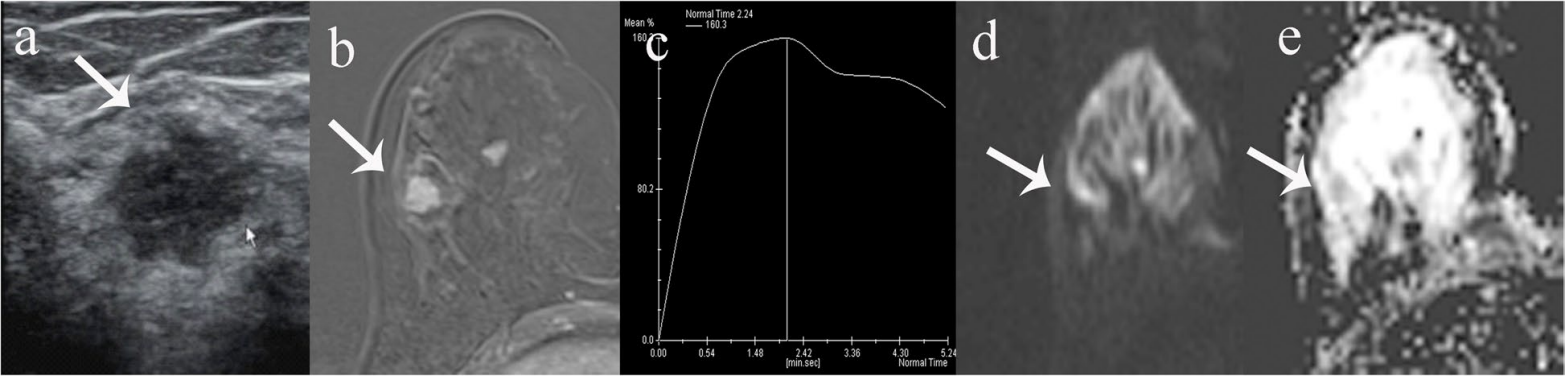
False-positive MRI finding for a lesion with US BI-RADS 4B category. a Gray-scale US revealed 13 mm irregular hypoechoic mass with indistinct margin on the right breast and 4B category was given. b, c Dynamic contrast-enhanced MRI showed an irregular mass with irregular margin and washout kinetics. d-e The lesion showed hyperintensity on DWI and hypointensity on ADC mapping, with mean ADC values of 1.11 × 10^–3^ mm^2^/s. BI-RADS 4 category was given on MRI. Pathology showed intraductal papilloma
